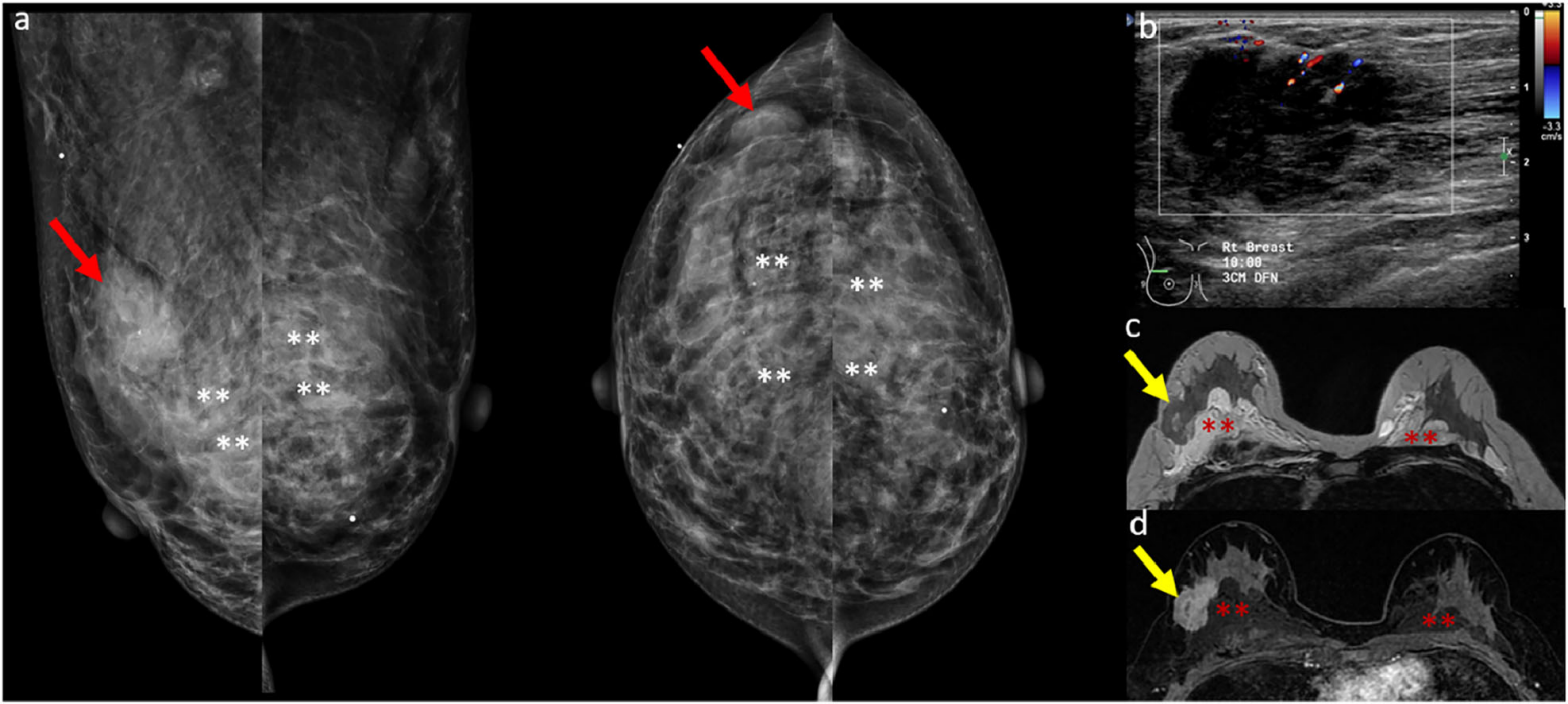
a MLO and CC mammograms in a 40-year-old woman revealed a focal asymmetry in the upper outer quadrant of the right breast (red arrows) adjacent to isodense polyacrylamide gel (white asterisks) previously injected for breast augmentation. b Ultrasound showed an irregular hypoechoic mass with indistinct margins and internal vascularity in the upper outer quadrant of the right breast that correlated with the mammographic abnormality. Imaging features were deemed suspicious, and a biopsy was recommended. c Axial T2-weighted non-fat-saturated and (d) post-contrast T1-weighted fat-saturated MRI images revealed an irregular enhancing mass (yellow arrows) in the upper outer quadrant of the right breast. Ultrasound-guided biopsy revealed grade 3 invasive ductal carcinoma. Bilateral non-enhancing, T2 hyperintense fluid collections in the retroglandular spaces (red asterisks) correspond to residual polyacrylamide gel from previous augmentation
